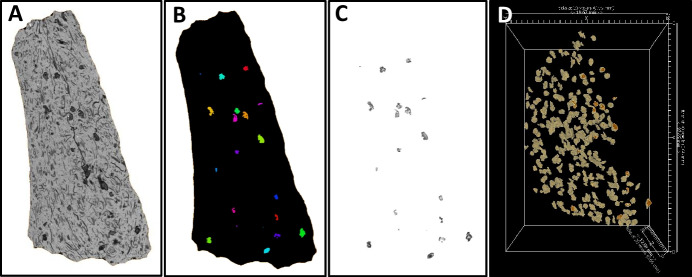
Segmentation process to separate rice spikelet bases from tomographic data of whole rice-tempered pottery sherd. A. 2D x-ray slice of sherd showing rice temper (lower x-ray attenuation) in black. B. Isolation and labelling of each rice spikelet base based on x-ray attenuation, size and shape. C. 2D slice of isolated rice spikelet bases with x-ray attenuation values. D. 3D rendering of rice spikelet bases from slice A-C (closest to camera) plus all spikelets bases in subsequent slices
